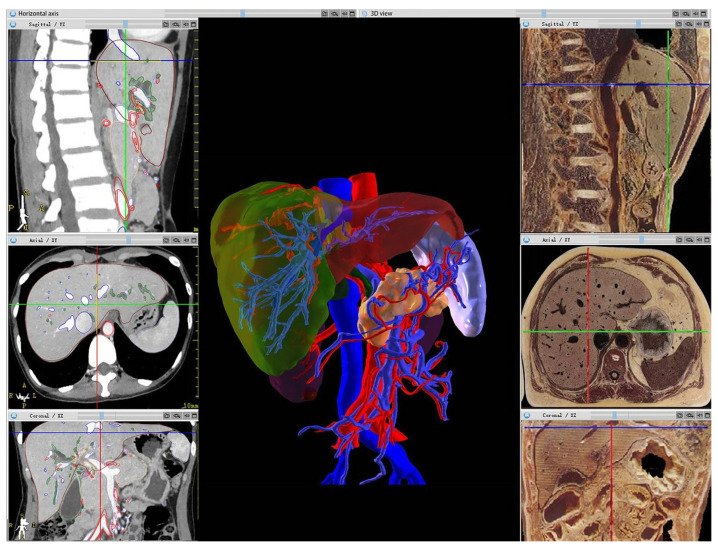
A liver CT scan is presented alongside its corresponding sectional anatomy. On the left side, we observe the liver CT image, while on the right side, we find a sectional anatomy representation. In the middle panel, a 3D reconstruction of the liver and its blood vessels, achieved through CT scanning, is presented [35].
